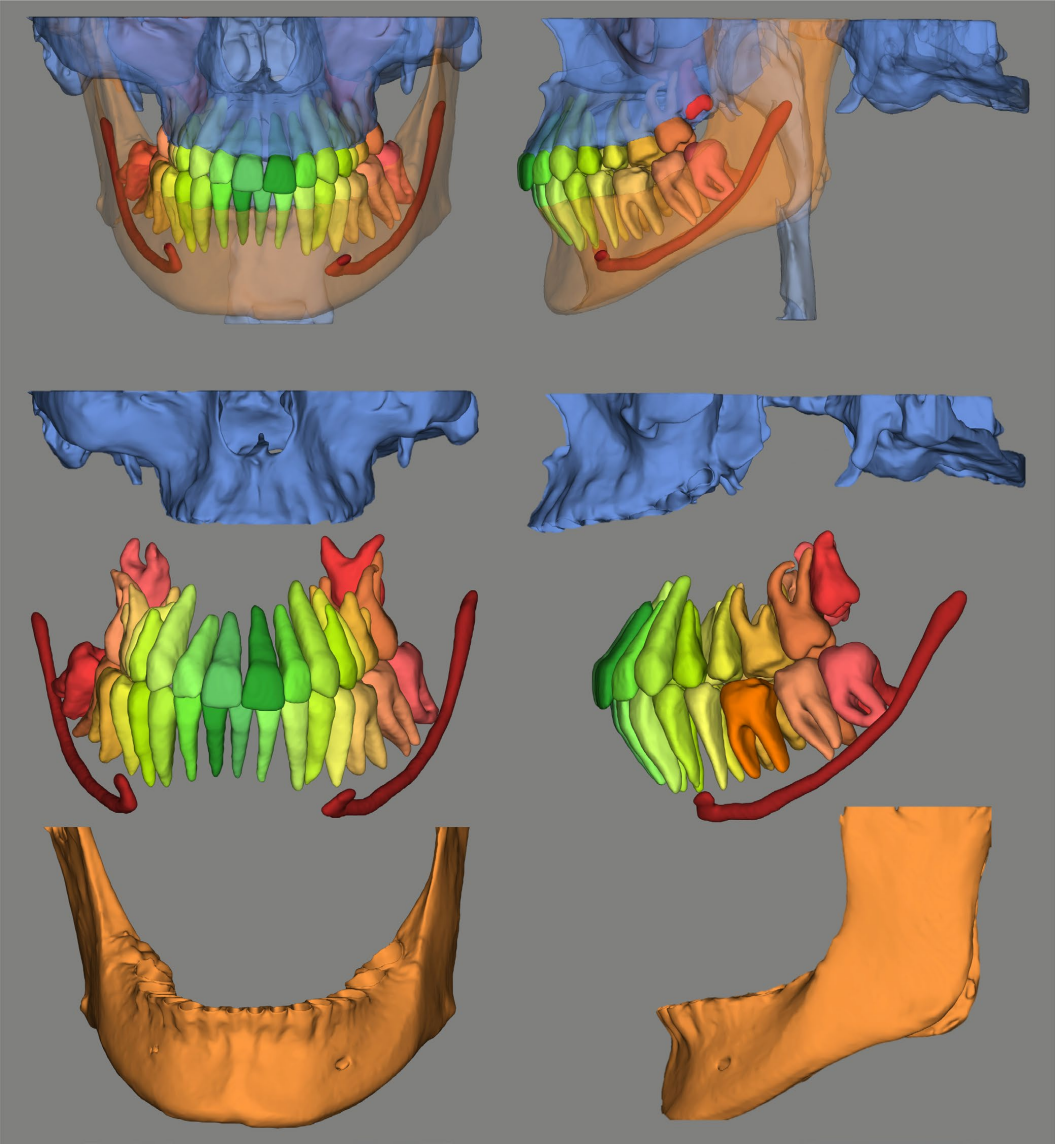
Segmentation of dentomaxillofacial structures from cone‐beam computed tomography data using the Relu online platform, presented as frontal and lateral views in both full and exploded views.
